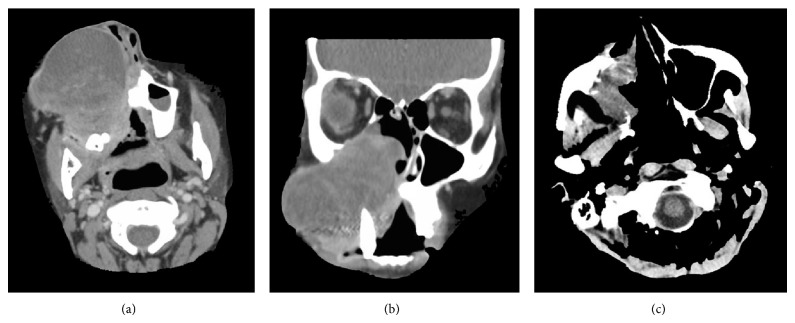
(a) A computed tomographic scan of the maxillofacial region with contrast showing the maxillary IMT in axial section at initial presentation July 2014. (b) A coronal section of the same CT scan highlighting extent of invasion. (c) A CT scan of the head without contract to rule out an intracranial hemorrhage October 2014. Image demonstrates tumor regression when compared with (a) and (b).
